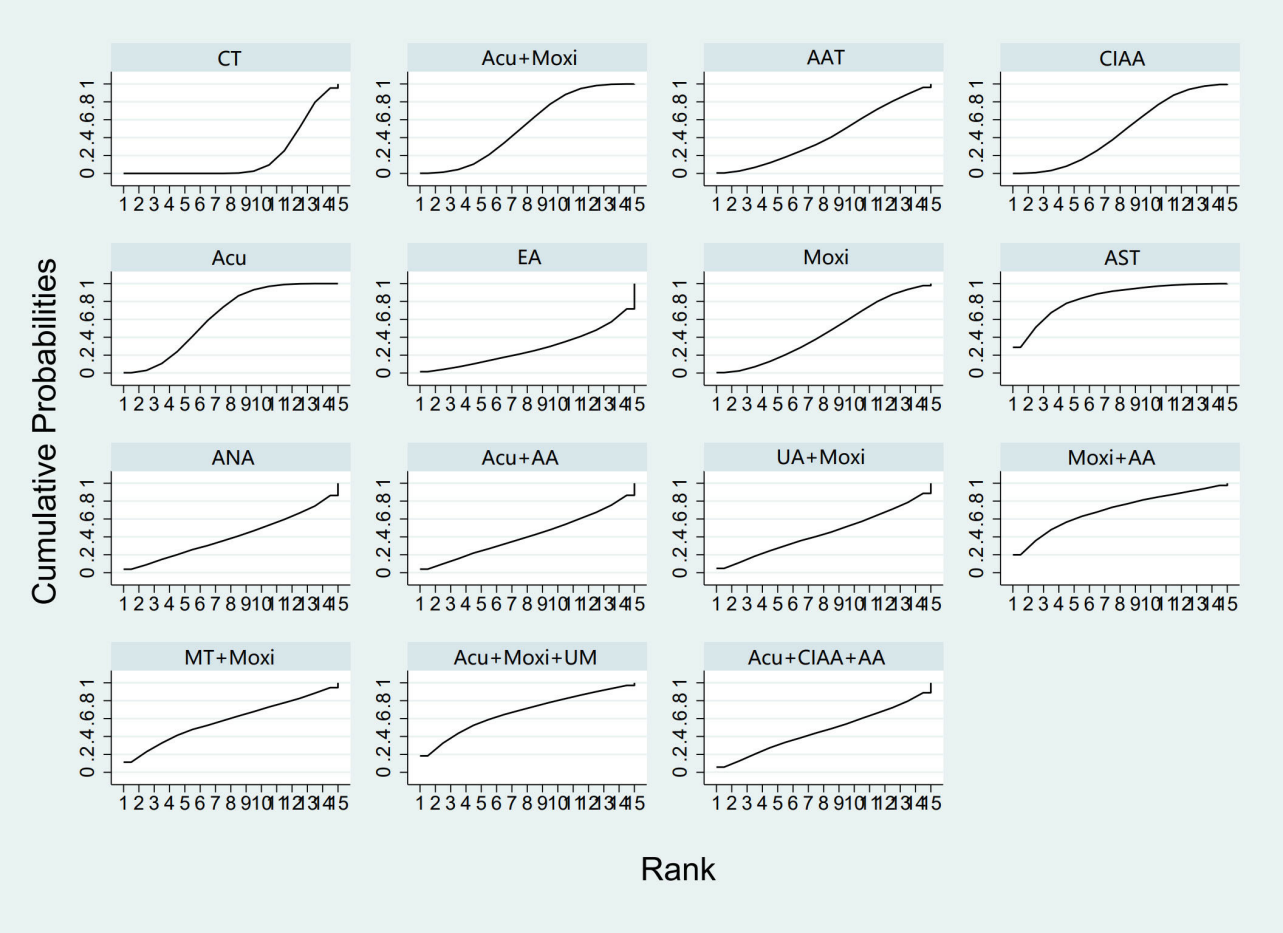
Efficacy ranking and cumulative probability graph of follicle-stimulating hormone (FSH) treated by different types of intervention. CT, conventional therapy; Acu, acupuncture; Moxi, moxibustion; AAT, abdominal acupuncture therapy; CIAA, catgut implantation at acupoint; EA, electroacupuncture; AST, auricular seed therapy; ANA, awn needle acupuncture; UA, umbilical acupuncture; AA, acupoint application; MT, massage therapy; UM, umbilical moxibustion.
